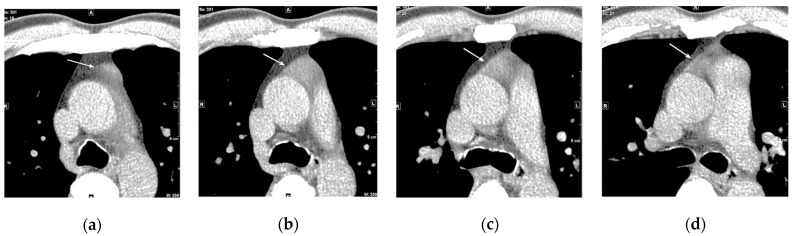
The incidental finding of soft tissue in the anterior mediastinum in a 53-year-old male. (a–d) Sequential axial low-dose computed tomographs demonstrating a homogeneous soft tissue density (arrows) in the prevascular space, raising the differential diagnoses of thymoma, thymic hyperplasia, or thymic remnant. It was interpreted as a thymic remnant. (A, anterior. P, posterior).
